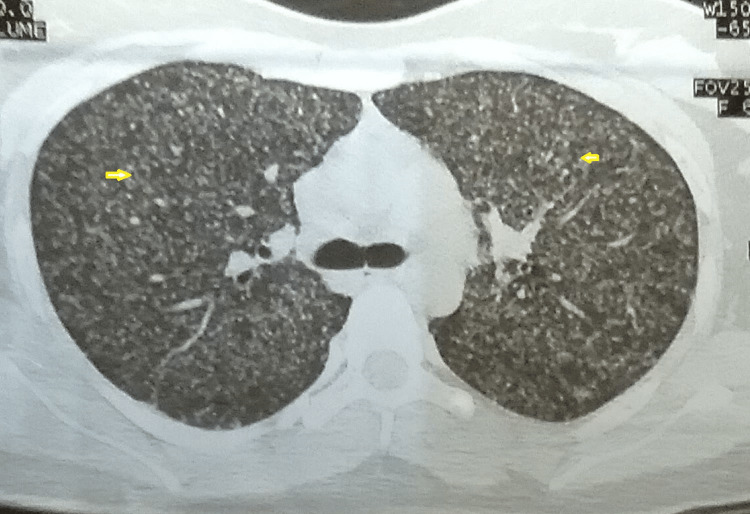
CT scan chest showing miliary mottling throughout both lungs (yellow arrow)
